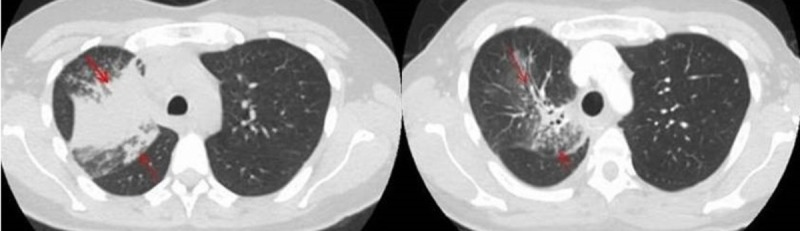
Representative computed tomography (CT) scan images of primary tumor at diagnosis and six months after initiation of erlotinib Left panel is axial CT scan image of the chest with primary tumor in the right upper lobe measuring 4.3 cm x 4.2 cm in size with lymphangitic spread. Right panel is the axial CT scan image of the chest six months after treatment initiation with erlotinib, the right upper lobe tumor now measures 2.4 cm x 2 cm in size consistent with RECIST 1.1 partial response. RECIST 1.1: Response Evaluation Criteria In Solid Tumors, version 1.1
